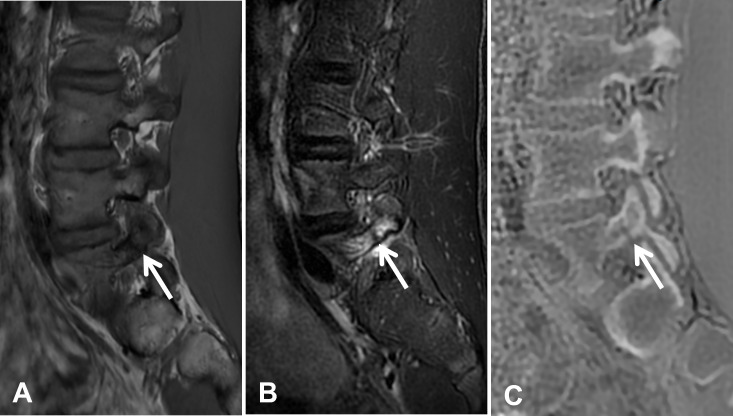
A 15-year-old male with a left L5 pedicle stress fracture. (A) Sagittal MR images demonstrate hypointense signal abnormality (arrow) on the T1-weighted image, associated bone marrow edema (arrow) on the fat-suppressed T2-weighted image (B) with a linear hypointense fracture line in the left L5 pedicle (white arrow). (C) The sagittal ZTE image clearly depicts the cortical discontinuity (arrow), confirming the diagnosis of a stress fracture.
